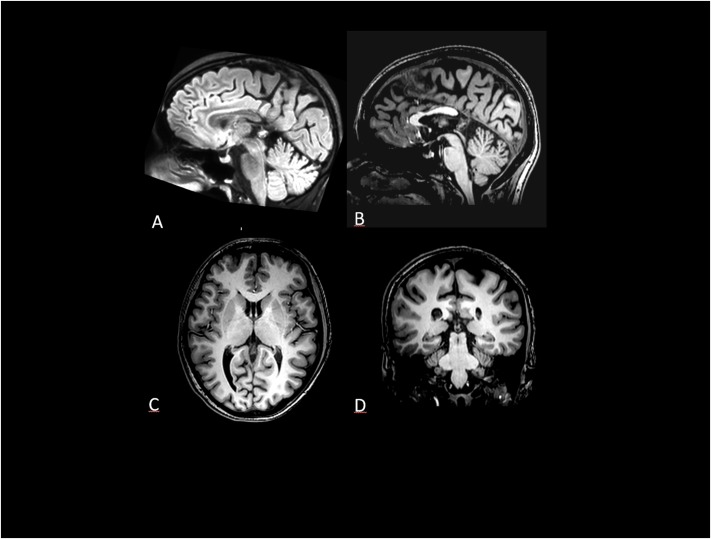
(A) Sagittal FLAIR 3D volume rendering and (B) Sagittal T1-WI shows normal rostrum, genu and body of corpus callosum and absent splenium (arrow) (C) Axial section through cavum septi pellucidi and internal cerebral vein within the cistern of the velum interpositum. (D) Coronal T1-WI: the retrothalamic cistern and medial wall of the lateral ventricles atri are delimited by cingulis and precuneus gyrus due to the splenium absence.
